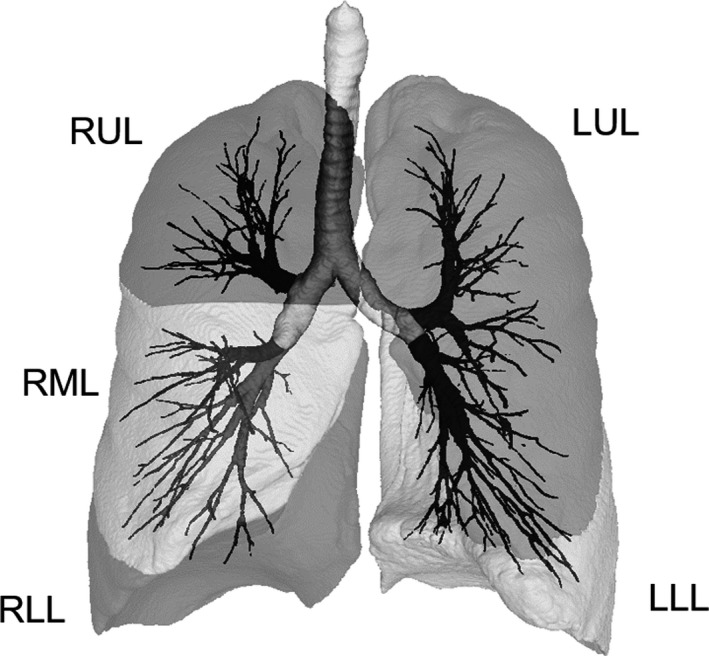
Methods used to calculate the airway tree to lung percentage ratio using CT. The airway tree and each lobe were segmented, and the volumes of tree portions in the right upper, middle, and lower lobes (RUL, RML, and RLL) and the left upper and lower lobes (LUL and LLL) were measured together with the lung volumes of the RUL, RML, RLL, LUL, and LLL. The airway tree to lung volume percentage ratio (AWV%) for each region was then calculated
